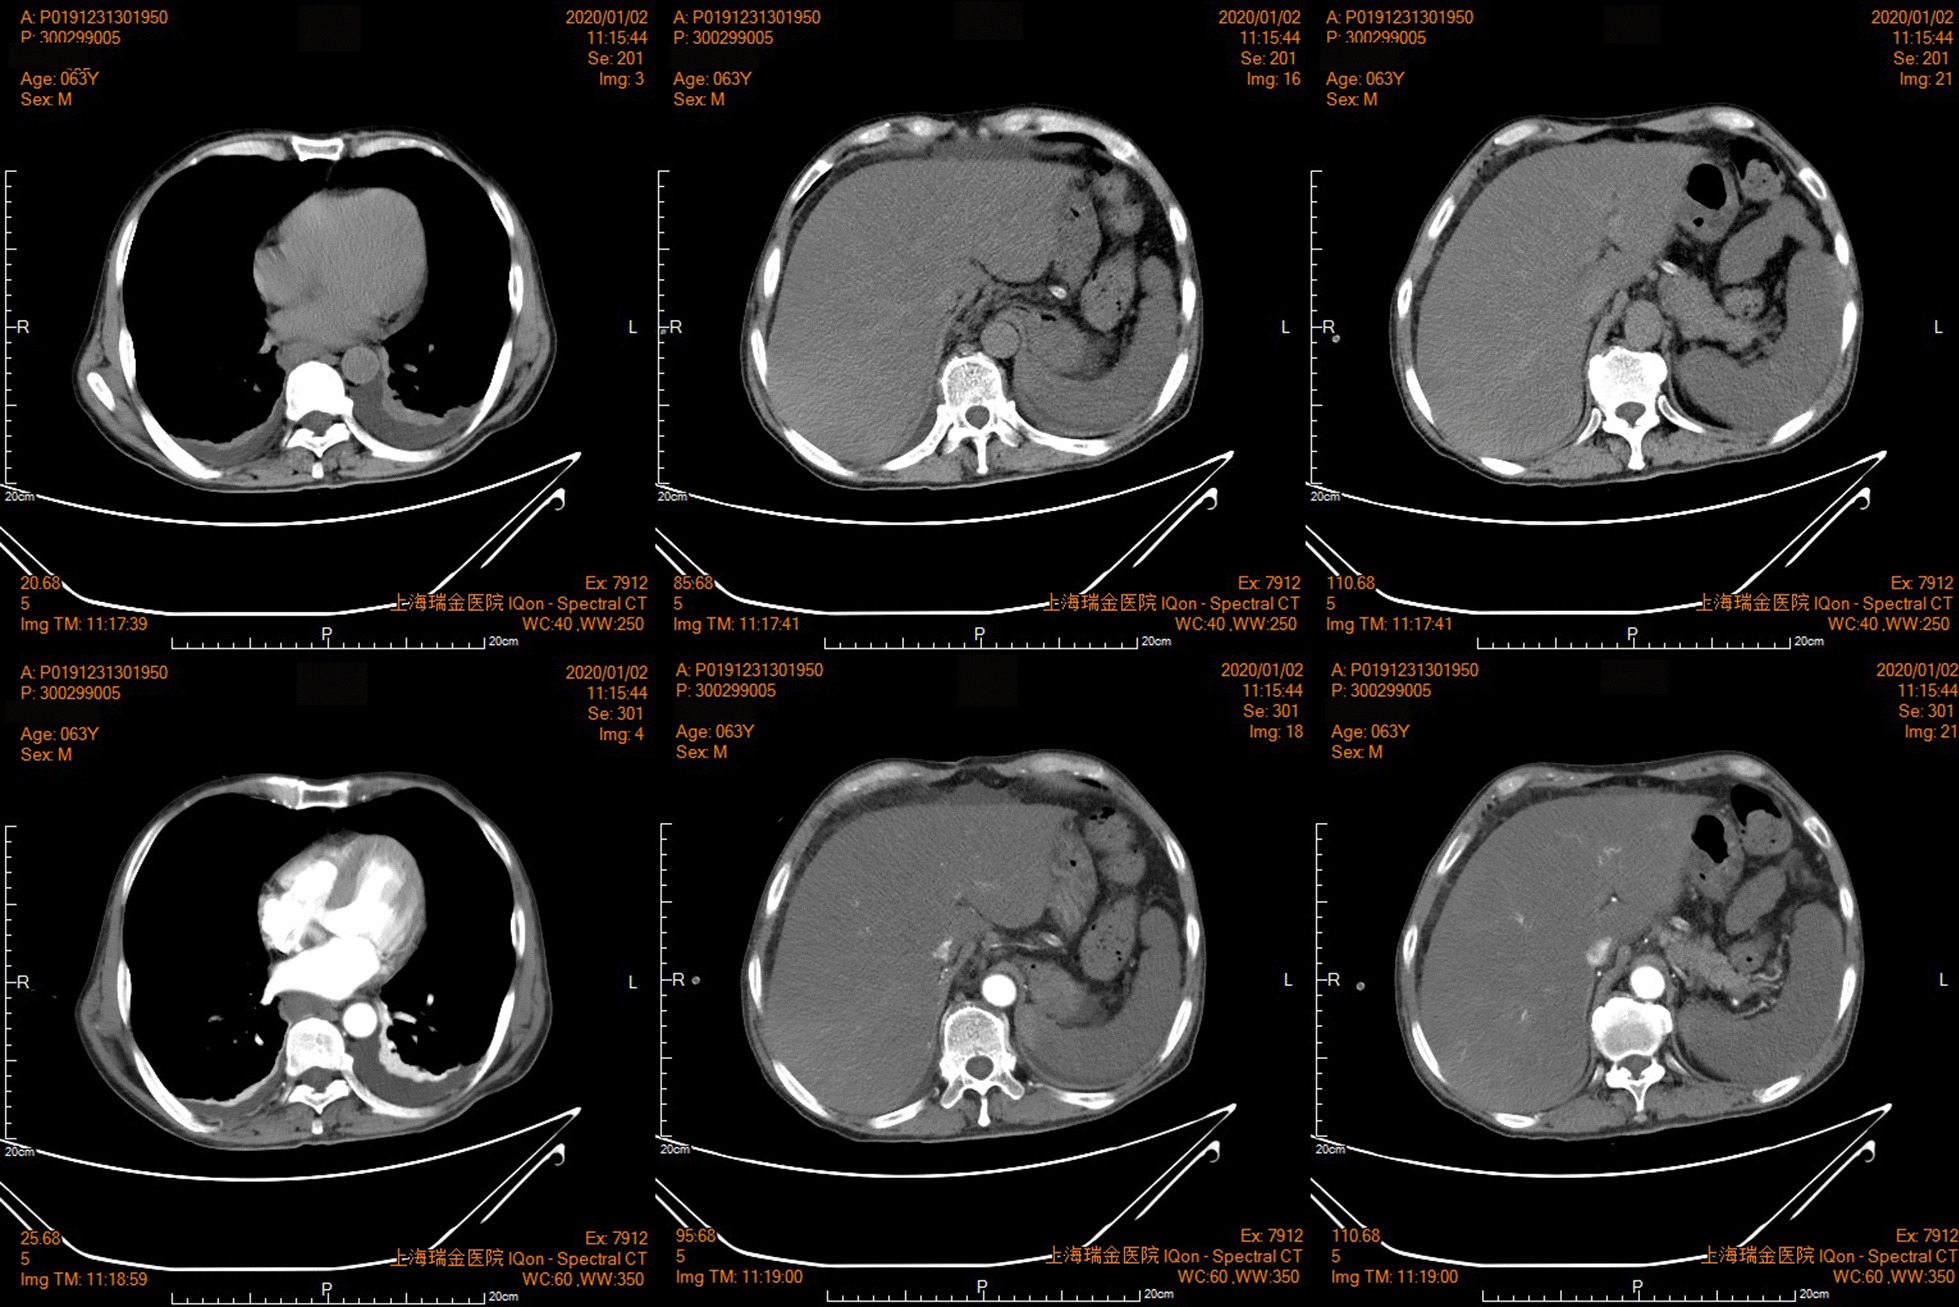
The CT image presenting abdominal pleural effusion, hepatomegaly and splenic infarction
